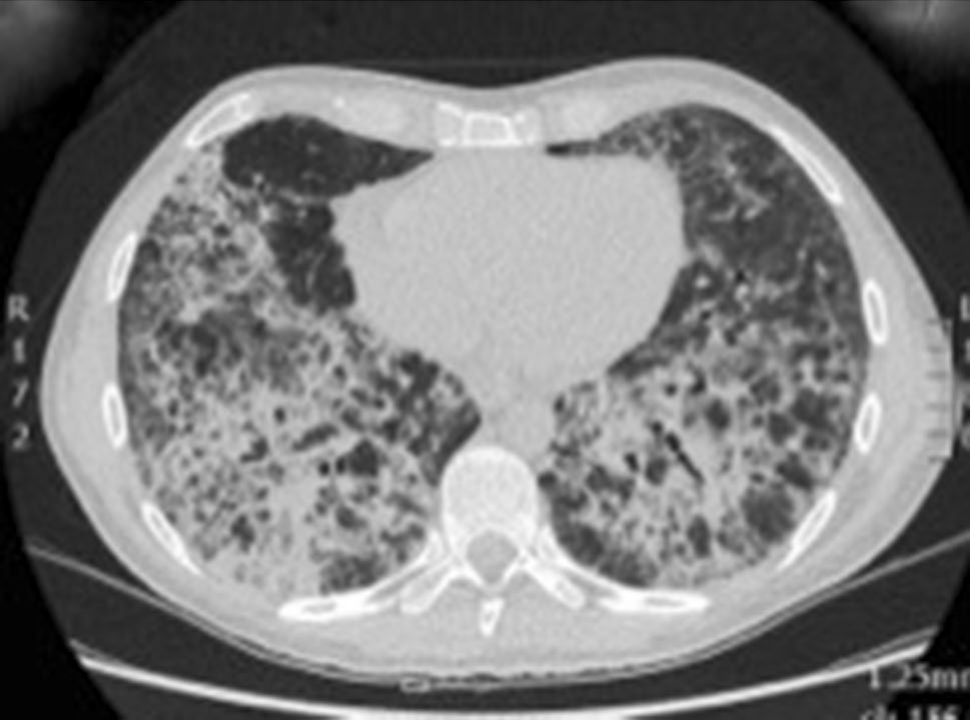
Chest CT after development of respiratory distress showing coarse interstitial thickening with cyst formation and areas of unaffected parenchyma suggestive of pneumocystis pneumonia, which was later microbiologically confirmed in BAL
